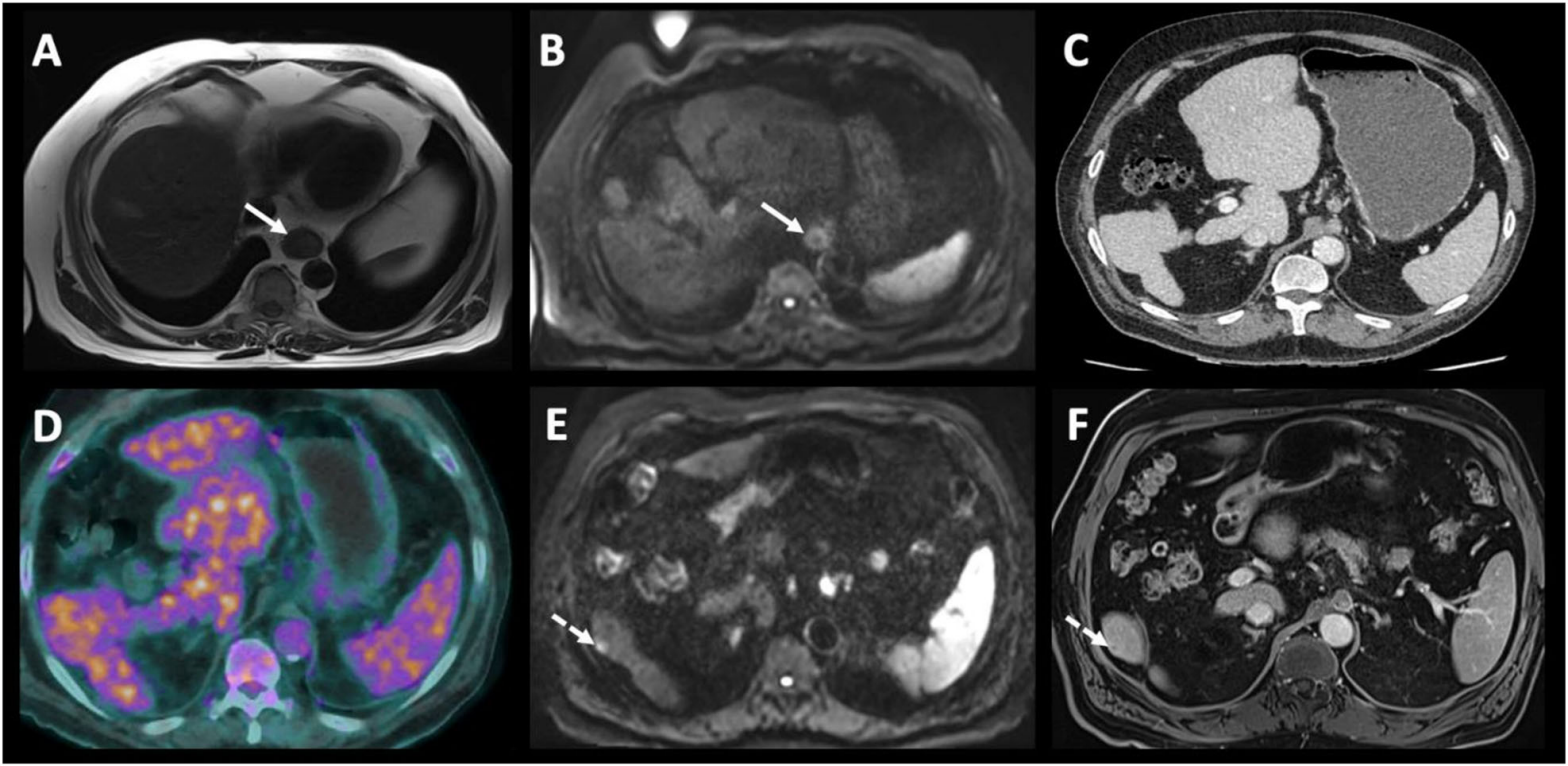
Example 4—Strengths of MRI for assessing liver metastases. Esophageal MRI evidenced the esophago-gastric tumor with intermediary signal on T2 (A, arrow) with restricted diffusion (B, arrow). The tumor was classified T3N2 based on MRI and echo-endoscopy (not presented). No metastasis was seen on CT and PET/CT (C, D). However, MRI evidenced a 7-mm liver lesion on segment VI with restricted diffusion (E, dotted arrow) and T1 hypo-intensity on portal venous phase (F, dotted arrow). Biopsy confirmed as a metastasis from esophago-gastric cancer
